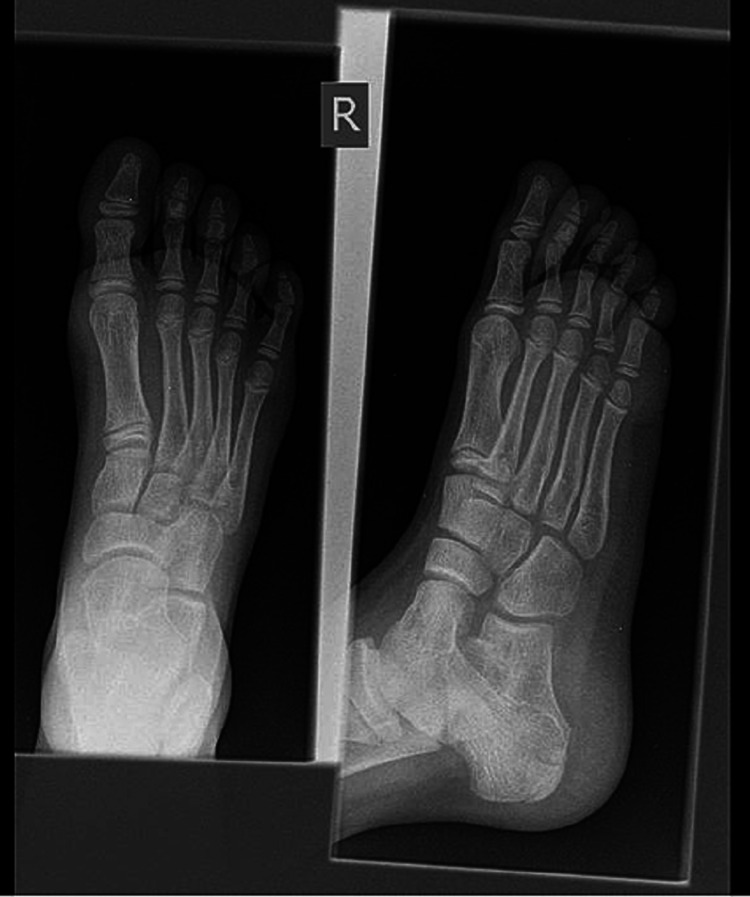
X-ray right foot AP and lateral view done at the time of original presentation having an inadequate view for proper diagnosis as the distal tibia is not visible
